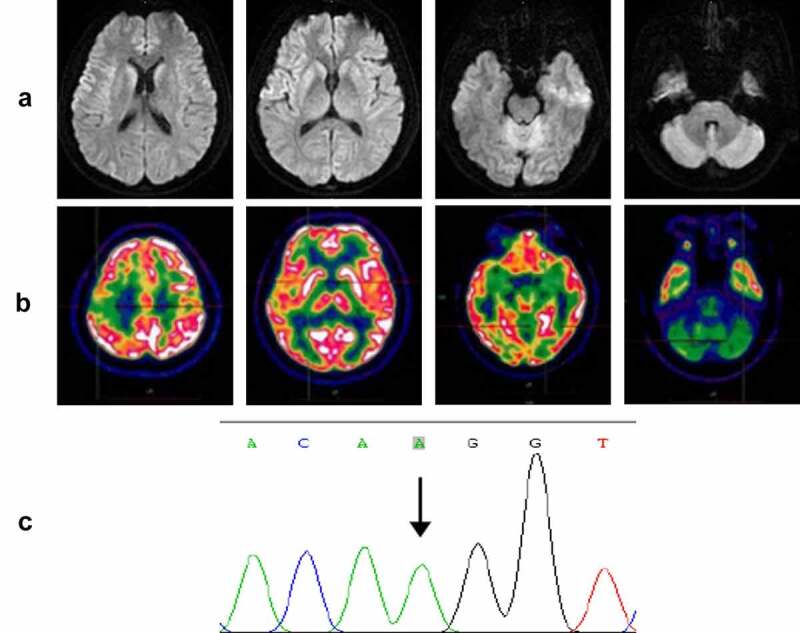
(a) Axial serial diffusion-weighted MRI showing restricted diffusion involving the right striatum, cerebellar cortex and cortical ribboning of the temporal and insular cortices; (b) ^18^Fluorodeoxyglucose-positron emission tomography showing severe glucose hypometabolism in the bilateral cerebellar cortex and mild glucose hypometabolism in the right frontoparietal cortex; (c) *PRNP* sequencing showing a homozygous substitution: c.563 C > A (p.T188K).
